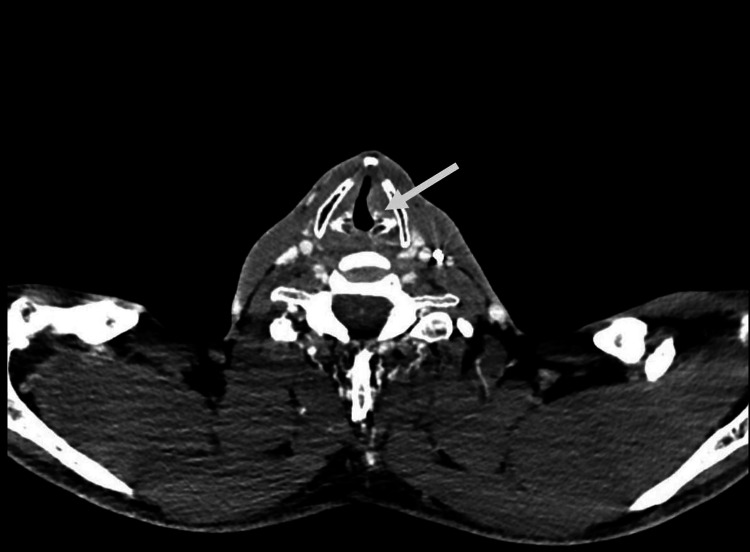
Patient's neck CT Medialization/slight rotation of the true left vocal cord (arrow sign) consistent with left vocal cord paralysis.
